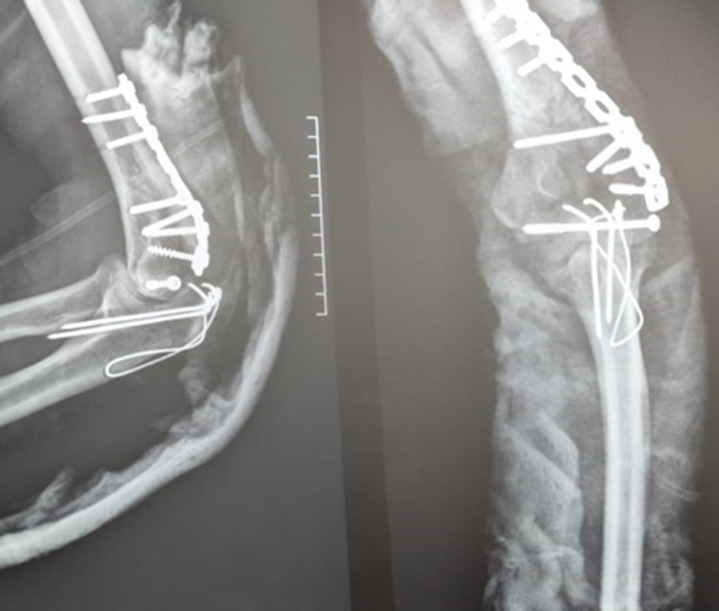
radiographie de contrôle post-opératoire d’une fracture type C1 après ostéosynthèse par une plaque postéro-latérale par voie postérieure trans-olécranienne
